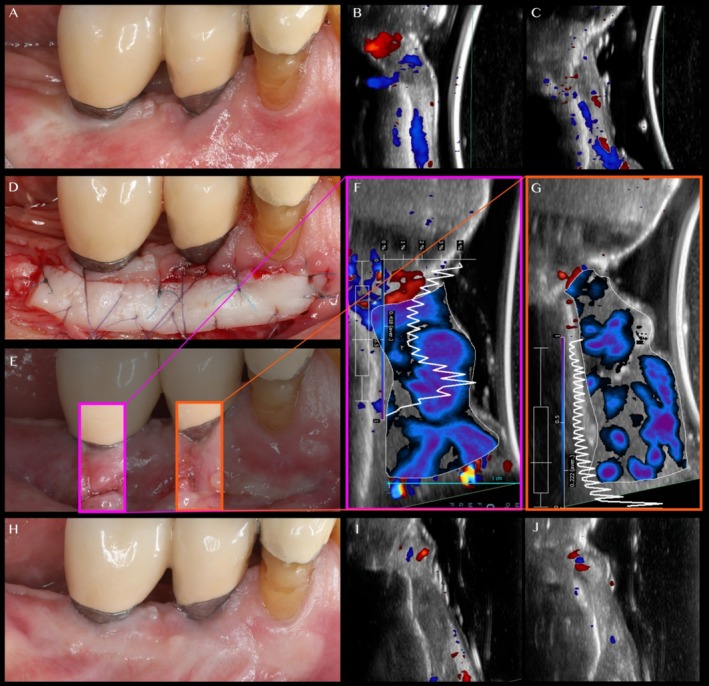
Ultrasonographic tissue perfusion and assessment of perfusion relief intensity (pRI) using the Color Doppler Velocity Mode (CDV). (A) Clinical view at baseline. (B and C) Baseline ultrasound scans of the implants #29 and #30 in CDV mode. (D) Surgical intervention involving APF + FGG. (E) 2‐week follow‐up. (F and G) Ultrasound scans of the implants #29 and #30 obtained in CDV mode at 2 weeks, with the concomitant assessment of pRI of the grafted area. (H) Clinical outcome at 12 months. (I and J) Ultrasound scans of the implants #29 and #30 in CDV mode taken at 12 months.
